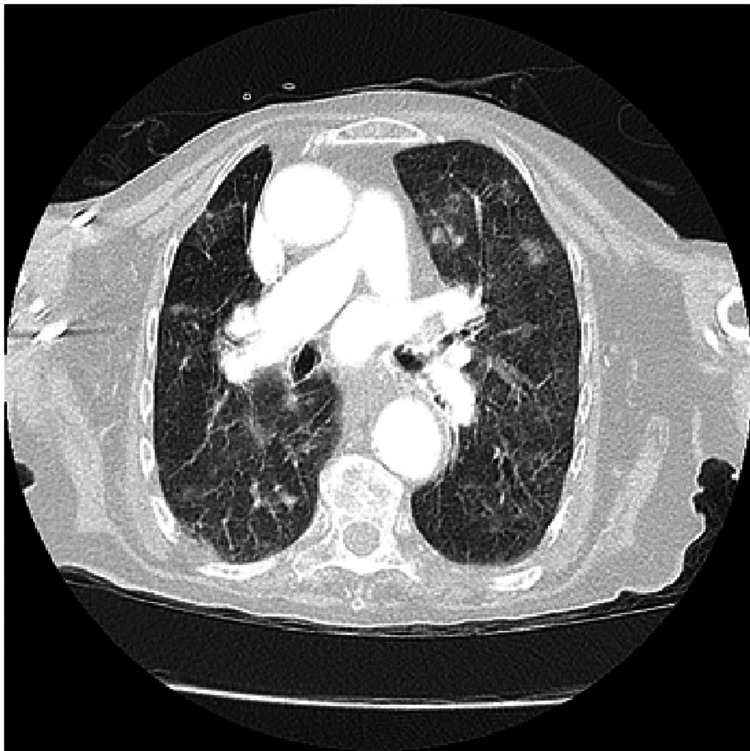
Axial CT chest on day four of admission, demonstrating widespread irregular, subcentimeter nodules with subtle ground glass peripheries suggestive of viral pneumonitis, but no frank consolidation.
